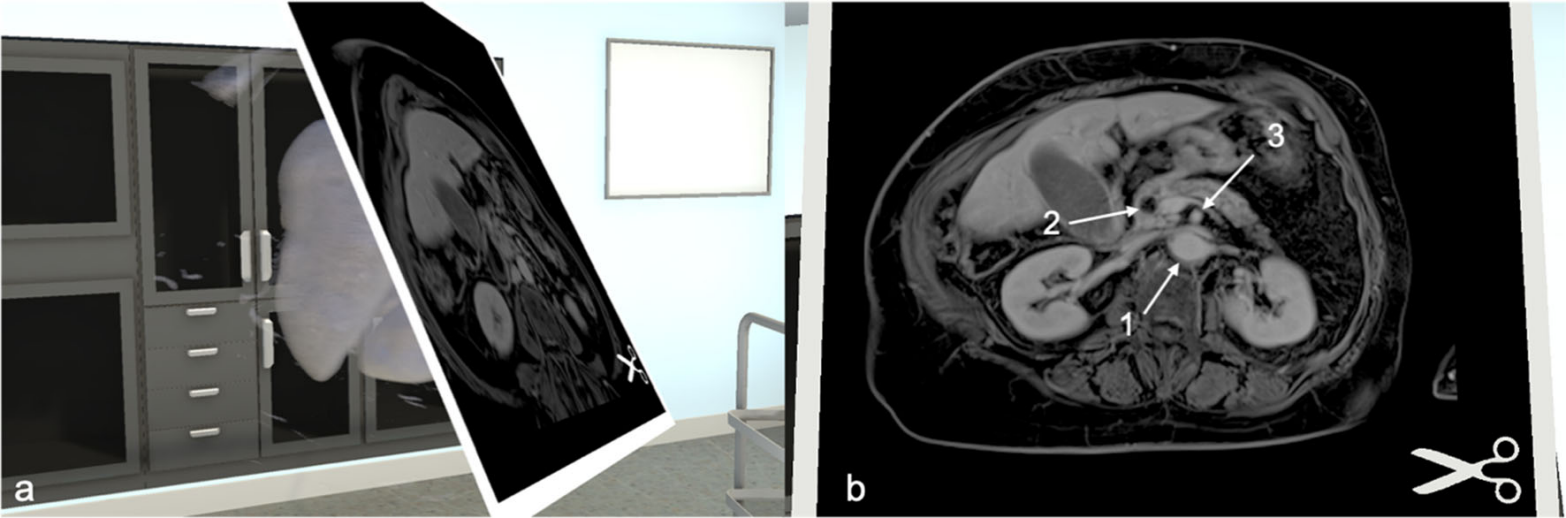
MR model of resectable pancreatic cancer. Similar to the CT model, the same assessment can be made for an MR model. a Section through the MR model of a patient with resectable pancreatic cancer. b Example of an axial section without visible tumour mass: (1) Aorta (2) Biliary duct (3) Superior mesenteric artery
